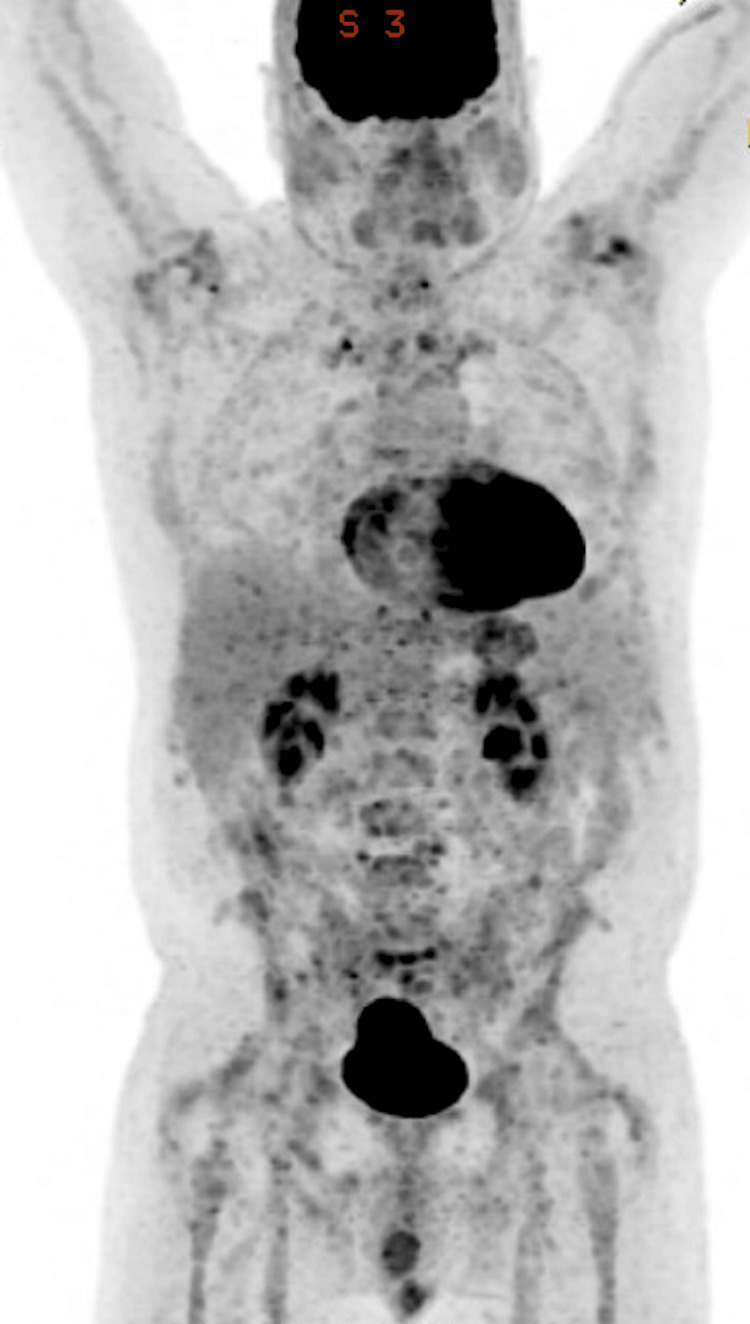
PET-CT findings after three cycles of epcoritamab and pirtobrutinib combination therapy Resolution of previous hypermetabolic lymphadenopathy and multifocal marrow and splenic lesions. Findings are compatible with complete response (5 PS = 1) PET-CT: positron emission tomography-computed tomography
